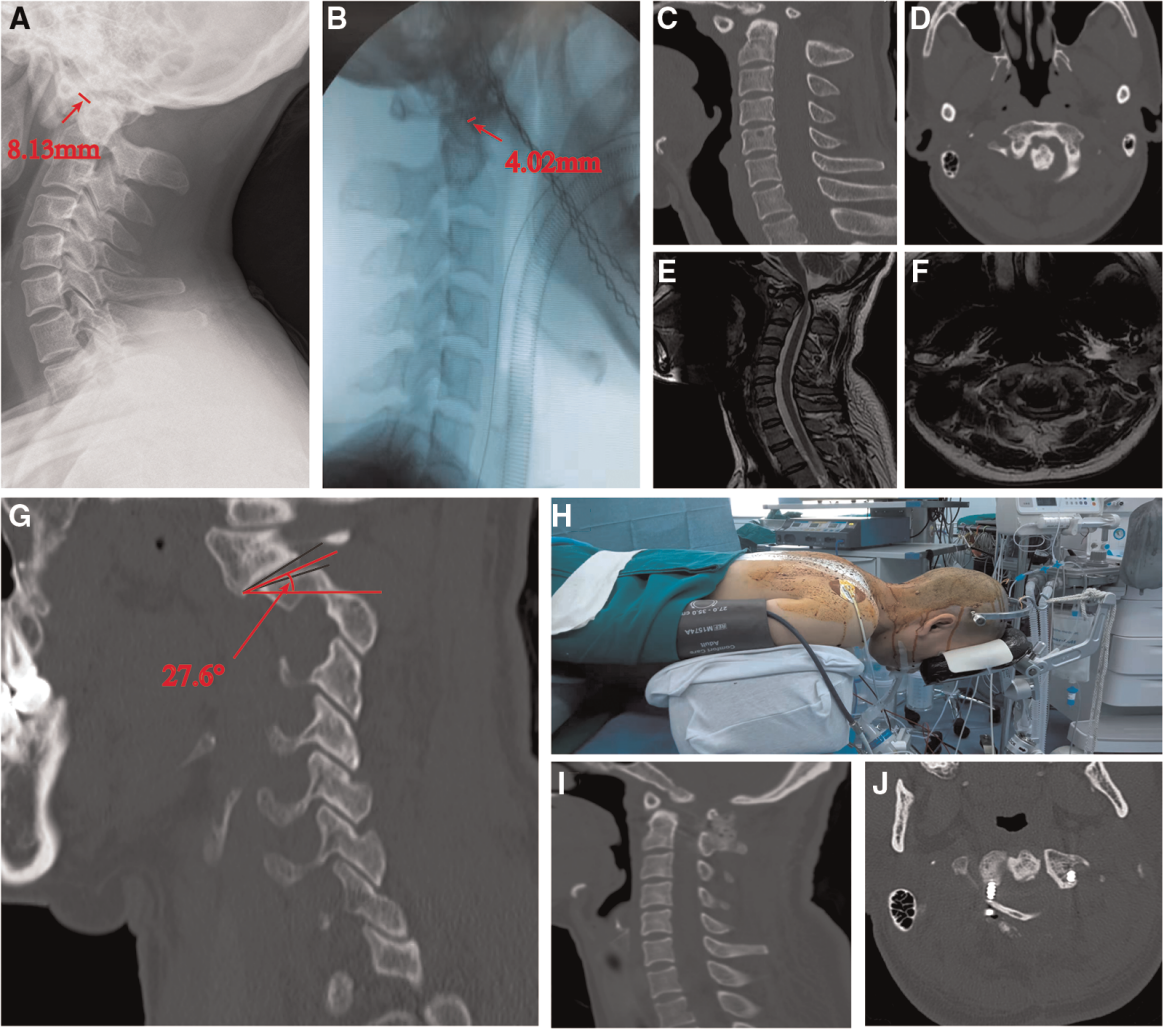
(A,B) A 42 years old female with AAD, traction was also conducted for her to evaluate the difficulty of reduction, which get the ADI before and after traction is 8.13 mm and 4.12 mm, RI is 50.55%. (C–F) CT and MRI showed that the fusion is not too much and the cervical spine is compressed severely. (H–J) The SAAJI was also measured, the result is 27.6° (G). We conducted a posterior operation with traction and get satisfied reduction.
